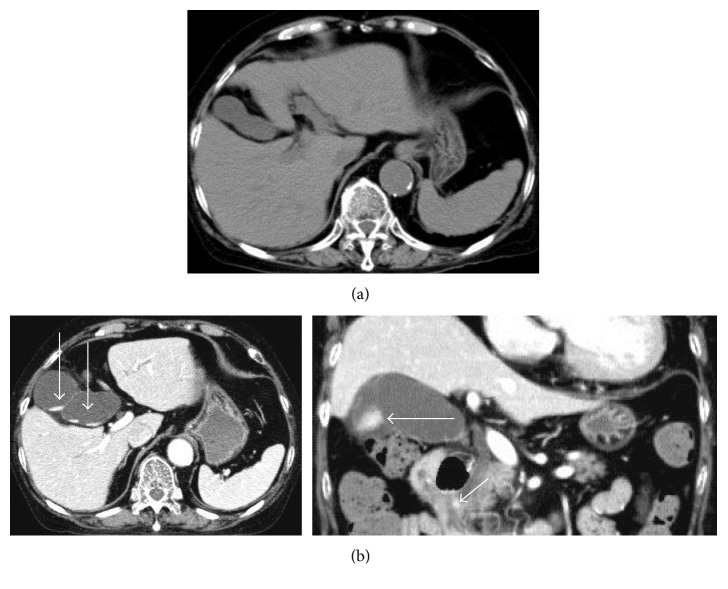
CT scans in Case 2. (a) Small stones or sludge were not detected in the biliary tract before administration of CTRX. (b) (*Left*, axial view; *right*, coronal view) small stones and sludge appeared in the gallbladder (long arrows) and common bile duct (short arrow) 10 days after ending CTRX administration.
